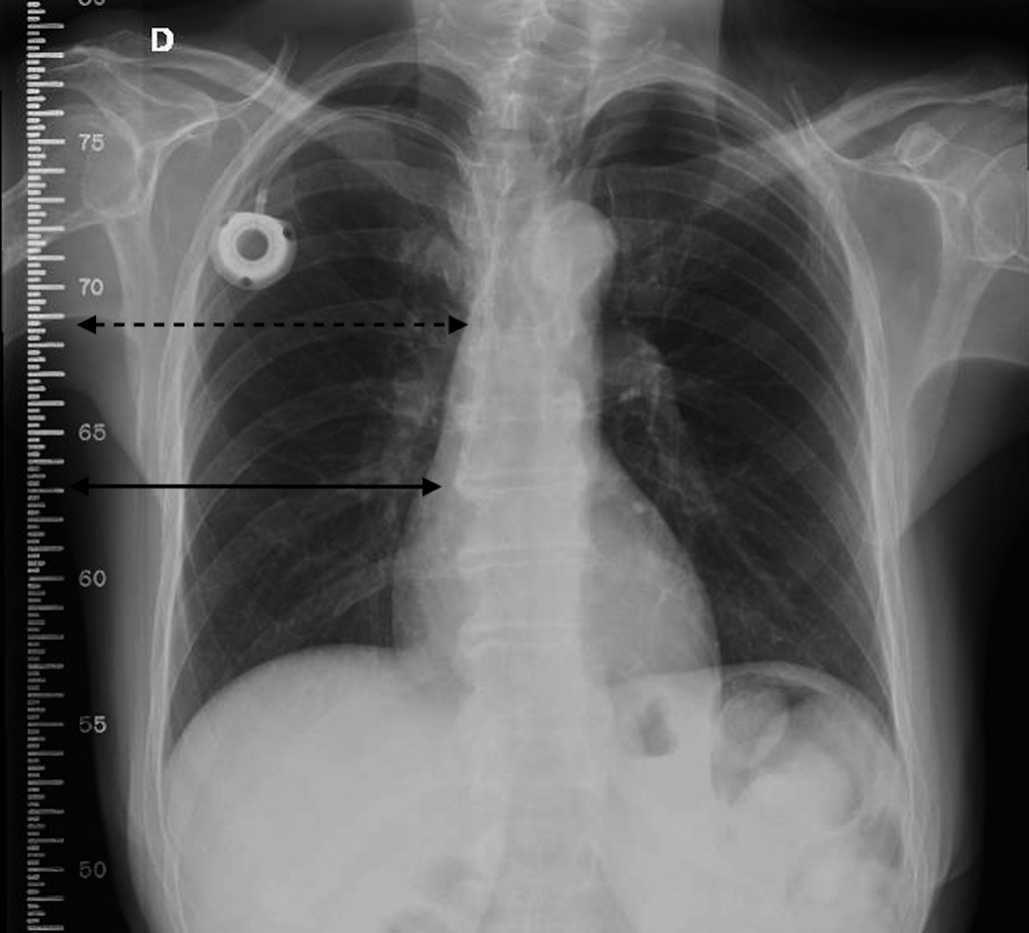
Postoperative standing X-ray image of the measurement of the catheter tip distance from the carina
